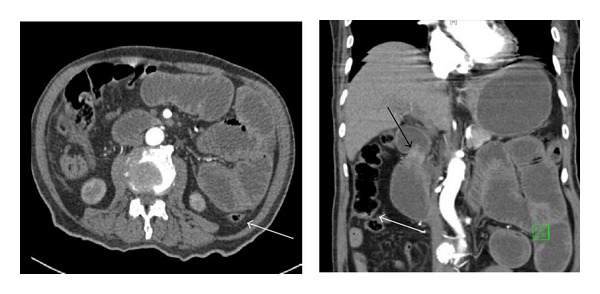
CT scan with oral and intravenous contrast showing dilated small bowel loops with edematous walls. Collapsed distal small bowel and colon is also noted (white arrow). A possible transition area in the small bowel loops was appreciated (black arrow).
